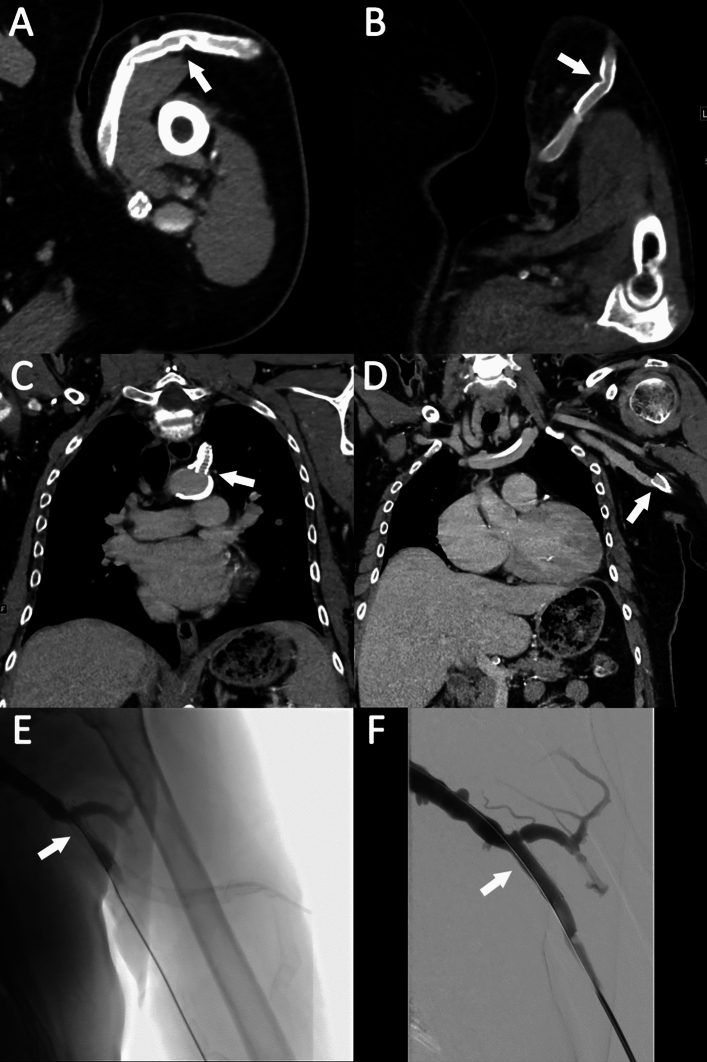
A 80 year-old male with history of multiple left upper extremity dialysis circuit vascular accesses, currently with malfunctioning left upper extremity axillary-axillary loop graft leading to poor flow rates during dialysis and inability to cannulate. CT angiography was performed to assess the current access and evaluate potential new surgical vascular access creation. CT angiography revealed multifocal stenotic disease of the graft within the cannulation zone (arrows) without evidence of complete occlusion (A and B). No central arterial stenosis was observed, but stenting of the proximal left subclavian artery was noted (arrow in C). However, CT angiography revealed a suspected focal high-grade stenosis of the left axillary venous outflow system just proximally to the previously placed stent graft (arrow) (D). At this point decision was made to pursue surgical revision with resection of the malfunctioning left upper extremity loop graft. However, given the venous outflow disease involving the left axillary vein, fistulogram was conducted along with endovascular treatment. The subsequent angiography revealed high grade in-stent stenotic disease (E). Extension of the stent graft was performed for endovascular reconstruction of the left upper extremity axillary venous outflow system in preparation for surgical revision of the existing left upper extremity axillary-axillary loop graft without evidence of residual stenotic on final digital subtraction angiography images (arrow in F)
